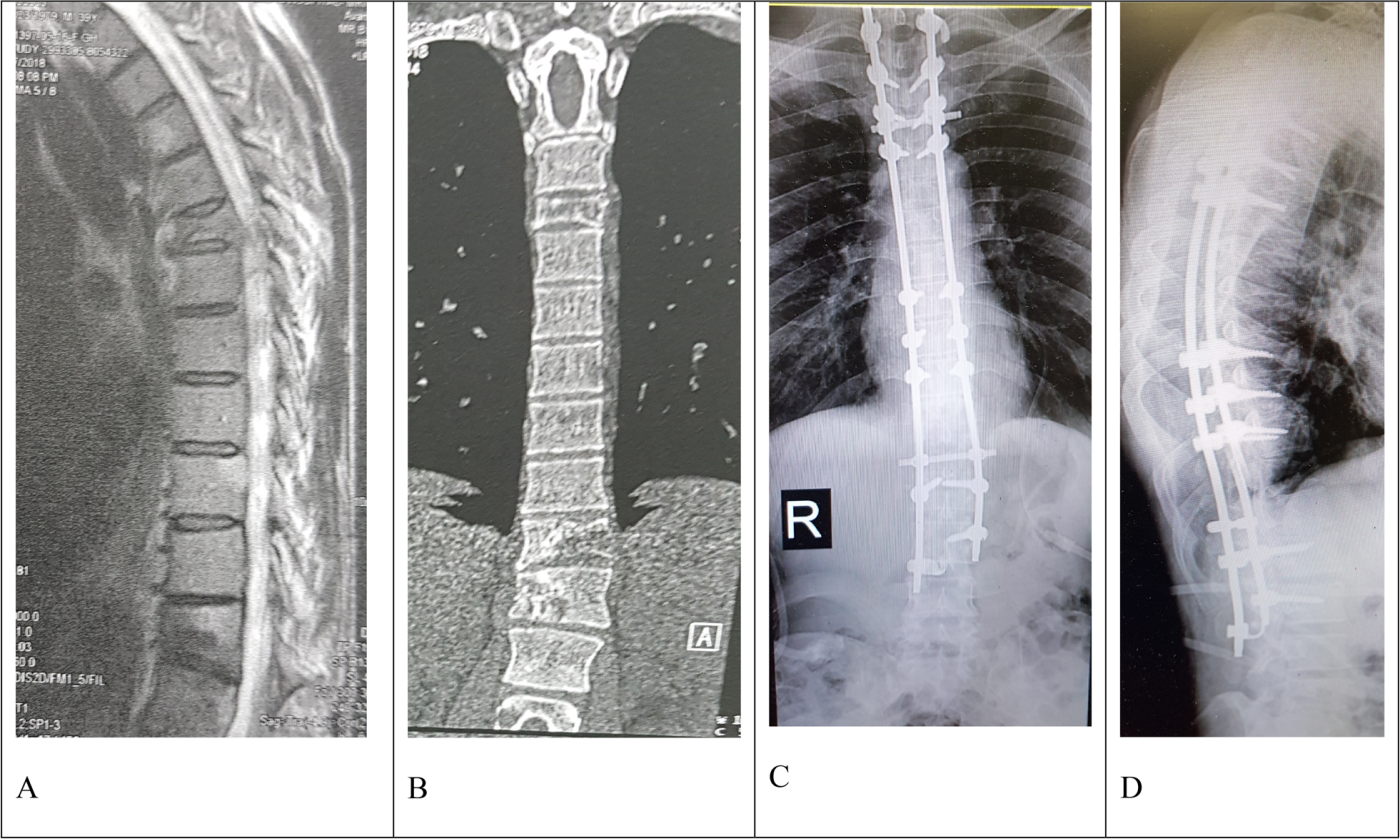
Spinal metastases from parotid adenoid cystic carcinoma in case 1. a, b Preoperative sagittal T2-weighted magnetic resonance imaging and coronal computerized tomography scan revealed multilevel significant bone destruction and pathological vertebral fractures. c, d Postoperative posteroanterior and lateral X-ray films
